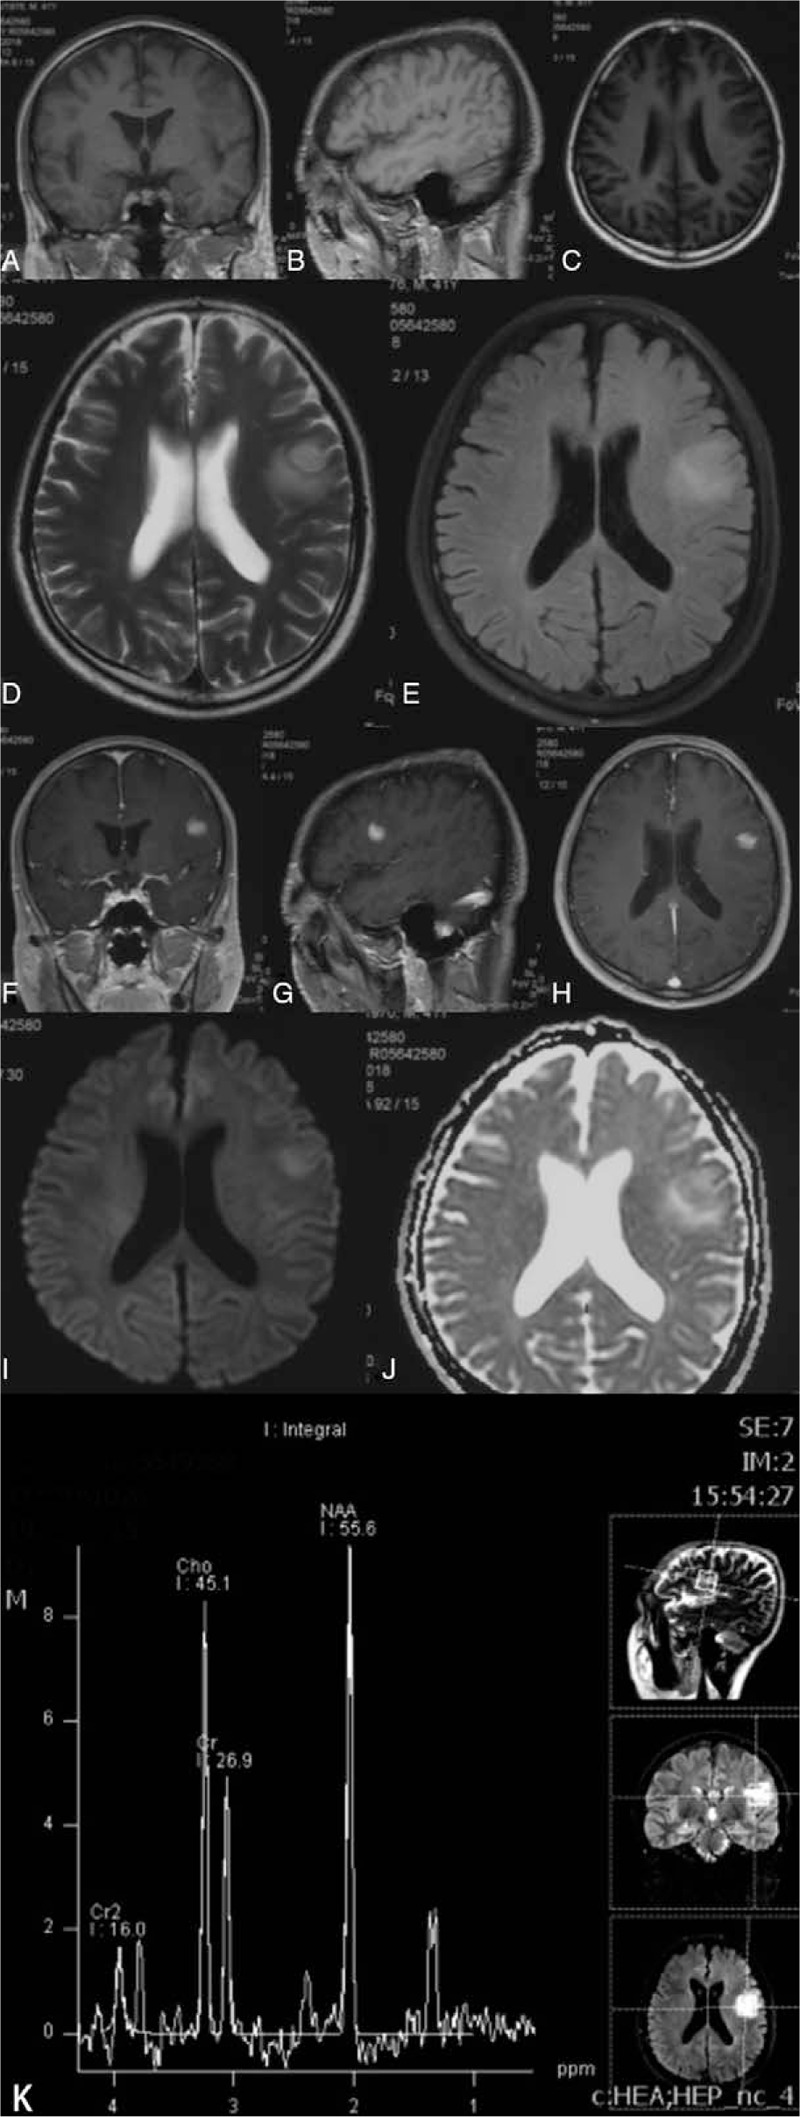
Preoperative MRI in Case 2. Coronal (A), sagittal (B), and axial (C) T1-weighted imaging showed a hypointense lesion in the left temporal lobe. The lesion showed hyperintensity with remarkable peritumoral edema on T2-weighted imaging (D) and FLAIR imaging (E). Coronal (F), sagittal (G), and axial (H) contrast T1-weighted imaging showed the lesion was markedly enhanced. The tumor showed iso- to hyperintensity with a circular hypointense core on DWI (I) and apparent diffusion coefficient imaging (J). MRS showed elevated Cho and NAA peaks and a decreased Cr peak (K).
